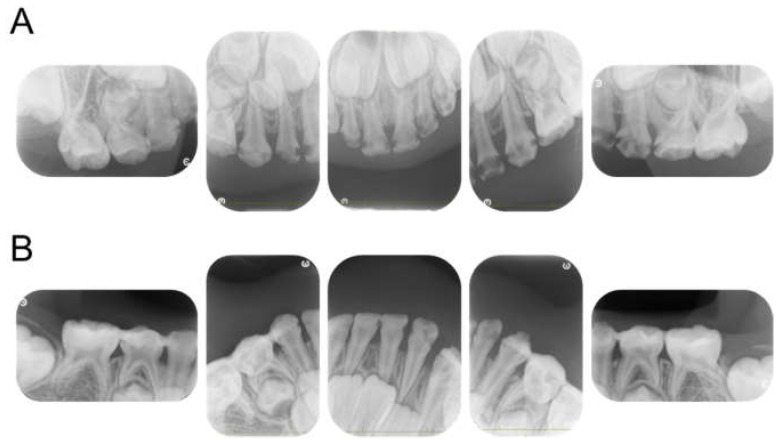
Periapical photographs. (A) At 4 years and 2 months. (B) At 4 years and 6 months.
